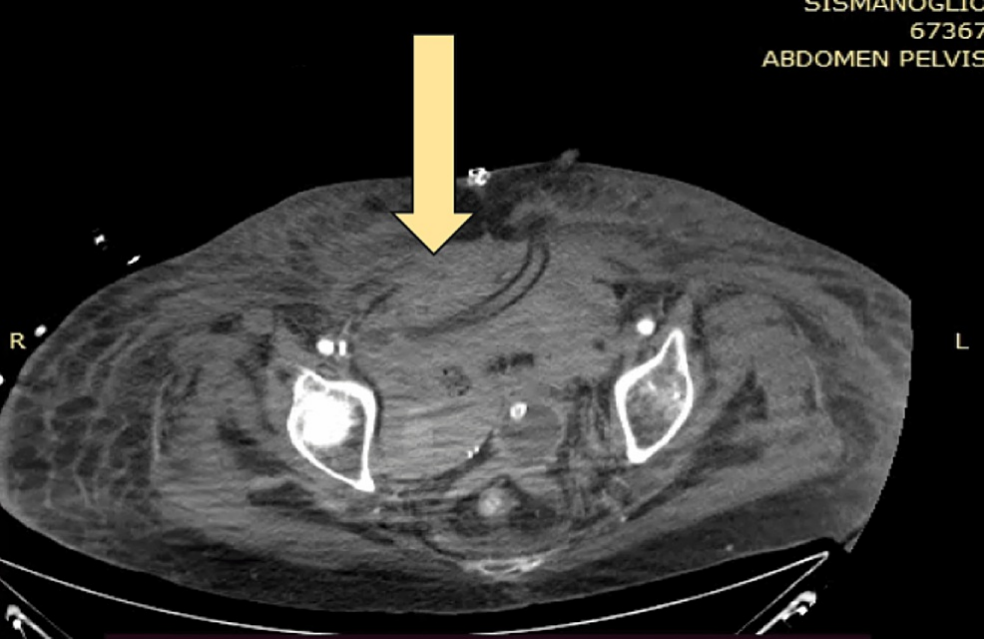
CT scan showing hematoma after the exploratory laparotomy (axial) CT: computed tomography
